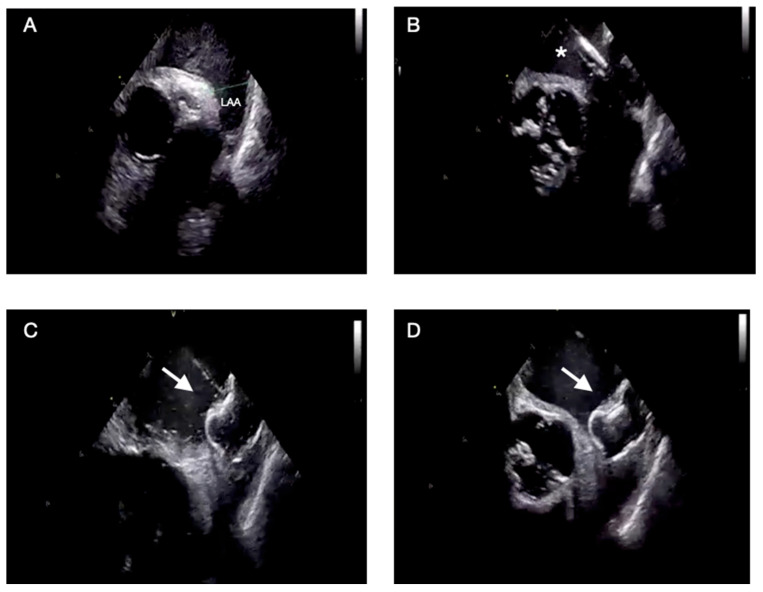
ICE-guided LAAO: procedural steps. (A) LSPV view with measurement of LAA dimensions to guide device sizing. (B) Delivery catheter (asterisk *) advanced into the LAA under ICE guidance in the left-atrial “home view.” (C) Partial deployment of the Occluder device (white arrow), still attached to the delivery cable. (D) Final device release (white arrow) with confirmation of stability and appropriate sealing of the ostial margins. Abbreviations: ICE: intracardiac echocardiography; LAA: left atrial appendage; LAAO: left atrial appendage occlusion; LSPV: left superior pulmonary vein.
